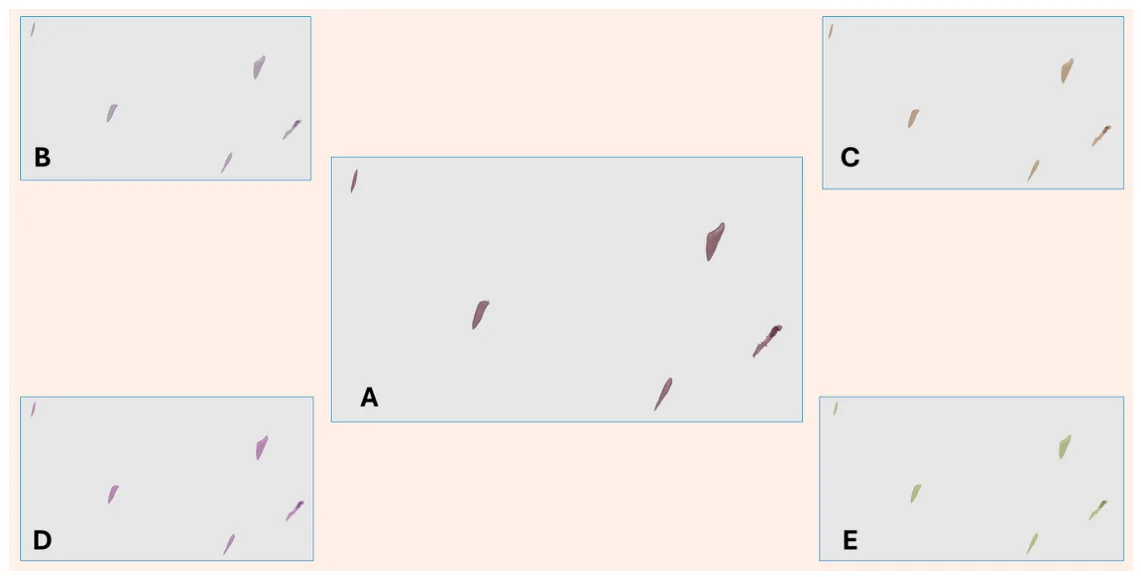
Representative combined visualization of canal systems obtained from CBCT acquisitions using the 3D-printed holder. (A) Combined visualization of all four acquisitions; (B) D0 vs. D5; (C) R0 vs. R5; (D) D0 vs. R0; (E) D5 vs. R5. Color coding: D0—blue; D5—orange; R0—red; R5—green. A high degree of spatial overlap is observed across all acquisitions, resulting in color blending due to minimal positional discrepancies.
